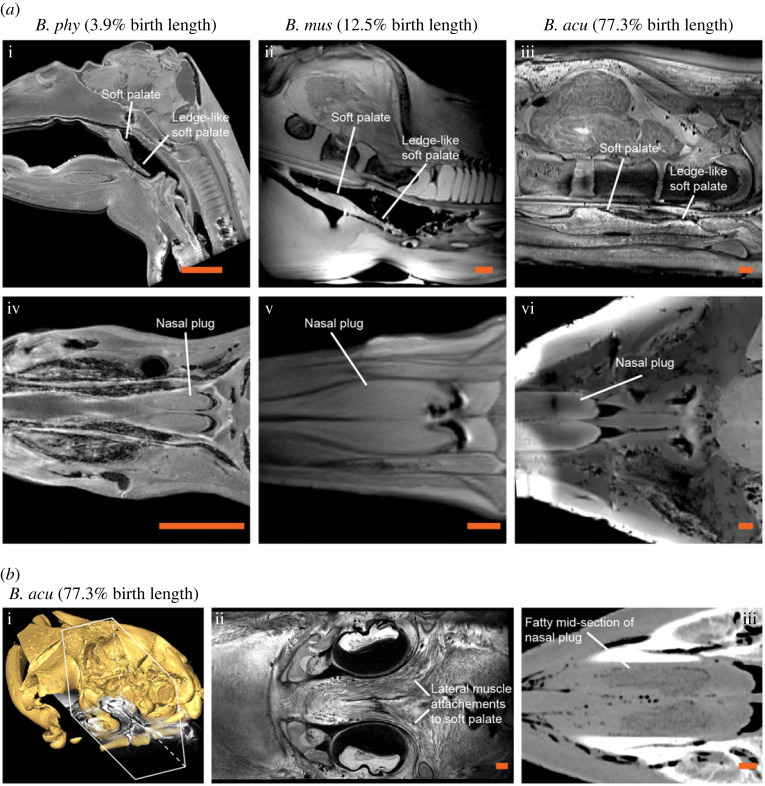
The developing soft palate and nasal plugs in rorquals. (*a*) Sagittal (i–iii) and coronal (iv–vi) magnetic resonance imaging slices through the head of an early fetal fin whale (*Balaenoptera physalus*, *B. phy*), a mid-fetal blue whale (*Balaenoptera musculus*, *B. mus*) and a prenatal minke whale (*Balaenoptera acutorostrata*, *B. acu*) showing the developing soft palate without tissue contrasts as indicative of a musculo-fatty oral plug (i–iii) and increasingly mature nasal plugs (iv–vi). (*b*) On (i), posterolateral view of digitally cropped (white box) minke whale skull from X-ray computed tomography surface reconstruction with co-registered coronal magnetic resonance imaging slice through the soft palate. In (ii), coronal magnetic resonance imaging slice (same as on (i)) through the soft palate showing muscle fibre attachment to the lateral sides of the nasopharynx. On (iii), coronal X-ray computed tomography slice through the nasal plugs clearly showing hypointense fatty mid-sections of the nasal plugs already formed. Scale bars represent 10 mm on all images.
